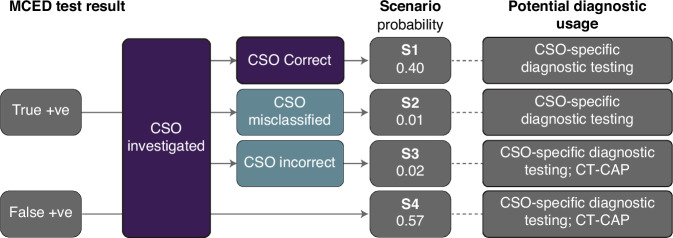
Decision tree model showing potential diagnostic resource use following cancer signal detected multi-cancer early detection (MCED) test results. ‘CSO misclassified’ means that the predicted CSO was incorrect, but the diagnostic test led to detection of a different cancer type. Probabilities are correct to two decimal places. CSO cancer signal origin, CT-CAP computed tomography of the chest, abdomen and pelvis.
